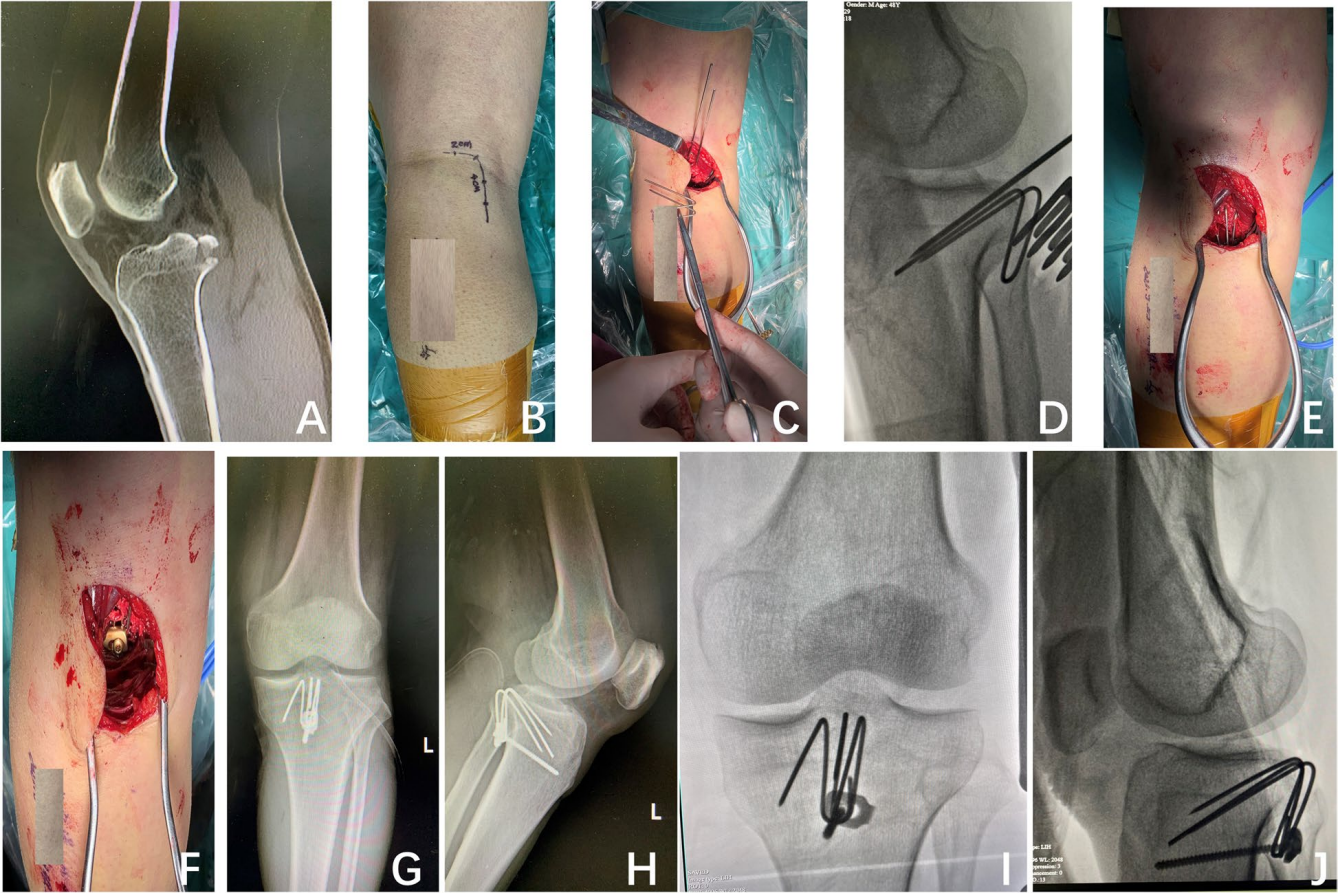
The case II presented with an acute knee injury, diagnosed as an avulsed left PCL tibial insertion. A Preoperative CT scan confirming the PCL avulsion fracture; B The planned inverted L-shaped postero-medial skin incision; C The first homemade pin-hook was implanted; D-E The second homemade pin-hook was implanted; F The pin-hook was fixed with a screw and a one-hole reconstruction plate; G-H Postoperative fluoroscopy showing the optimum position of the homemade pin-hook and fracture reduction; I-J Three-month postoperative follow-up radiographs showing fracture union and optimum position of internal fixation
